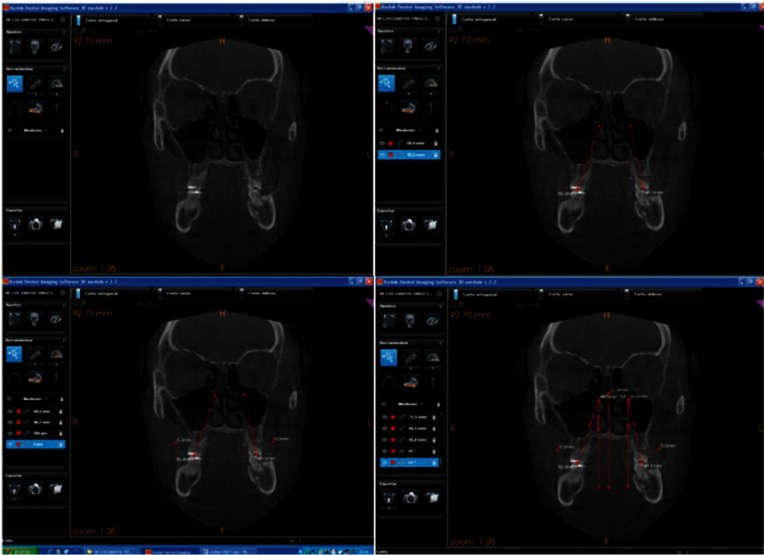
Angular measurements on Cone Beam method.
